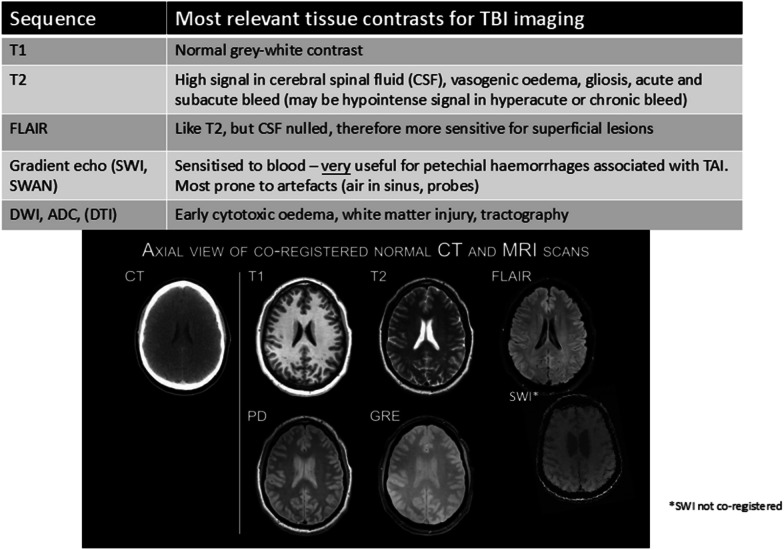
Clinical magnetic resonance imaging sequences: examples of magnetic resonance imaging sequences commonly used for traumatic brain injury
